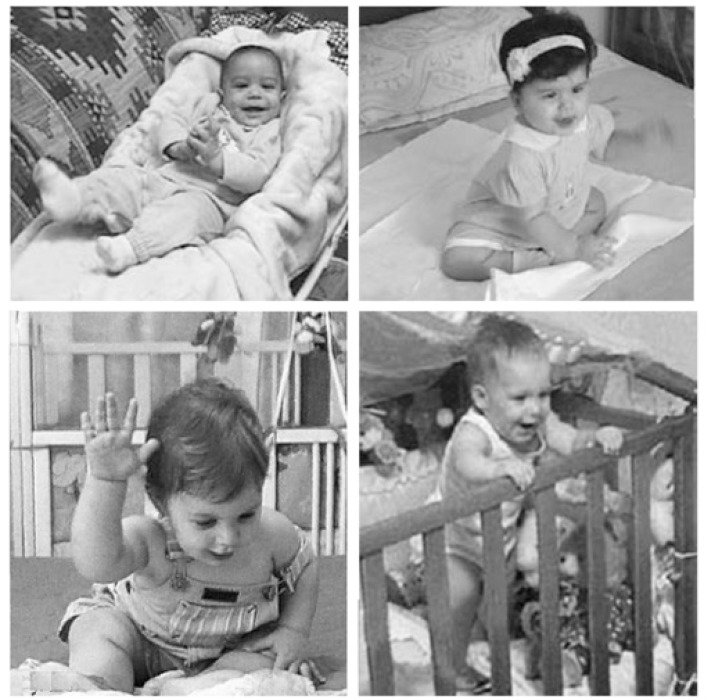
Katerina Mazokopaki’s baby dancers: Upper Left: Georgos, 3.5 months lying on a comfortable bed, responds with a big smile and gestures of hands and feet to accompany the music. Upper Right: Katerina, 9 months, smiles, bounces and extends her arms to ‘fly’ into action. Lower Left: Panos, 9 months, smiles his greeting, then beats the floor rhythmically with his right hand. Lower Right: Anna, 10 months, standing in her cot, smiles and starts dancing vigorously, swinging her bottom. All four sing with the music (Photos supplied to the CT).
